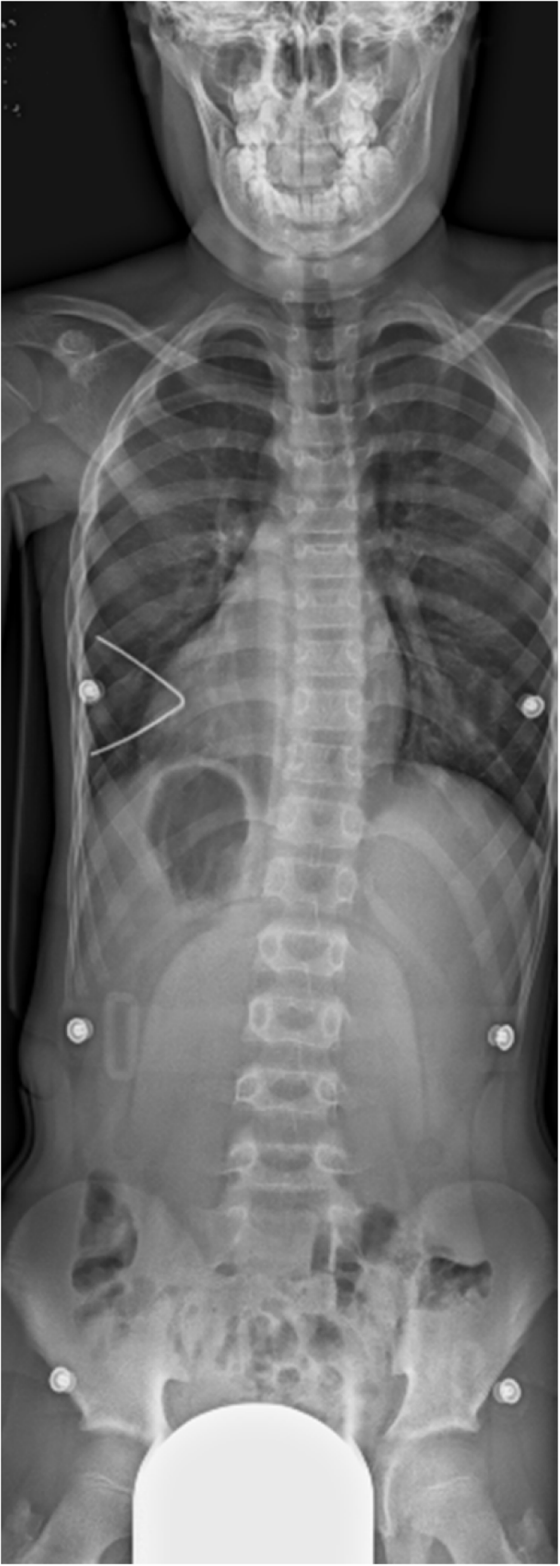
Radiograph wearing underarm brace. At treatment commencement, in spite of slight overcorrection, sufficient scoliotic curve correction by the brace was verified
